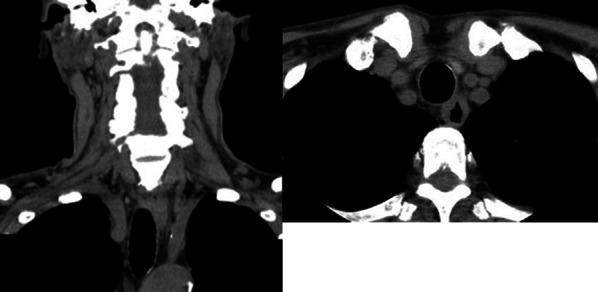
Postoperative CT. There were no remaining mediastinal foreign bodies
